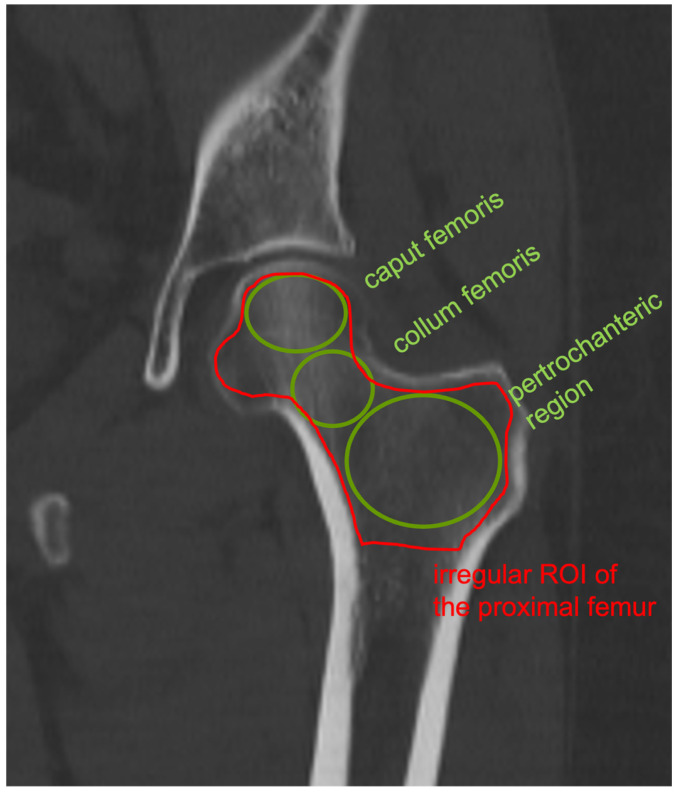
Coronal CT image of the left hip with correspondingly marked ROIs in the proximal femur.
